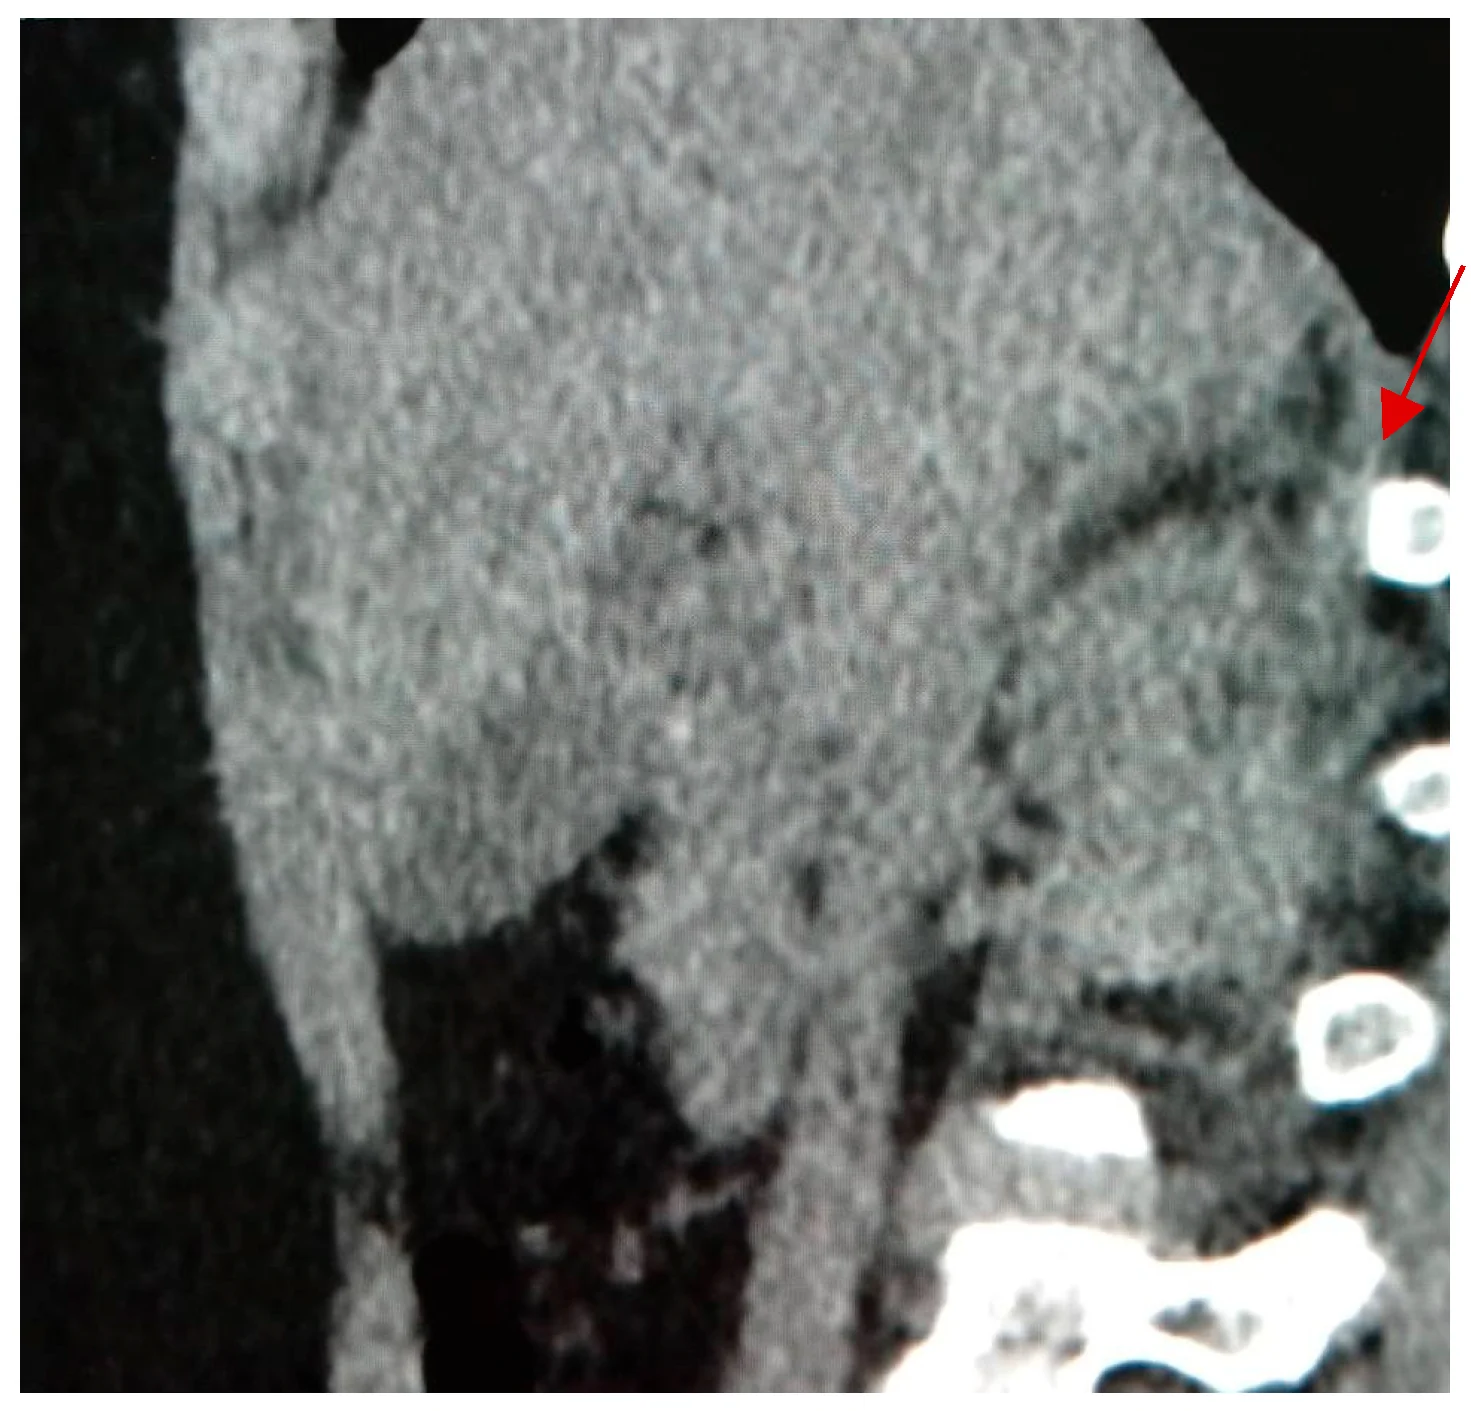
CT image showing a small fundal outpouching of the gallbladder (red arrow), highly suggestive of a gallbladder diverticulum.
